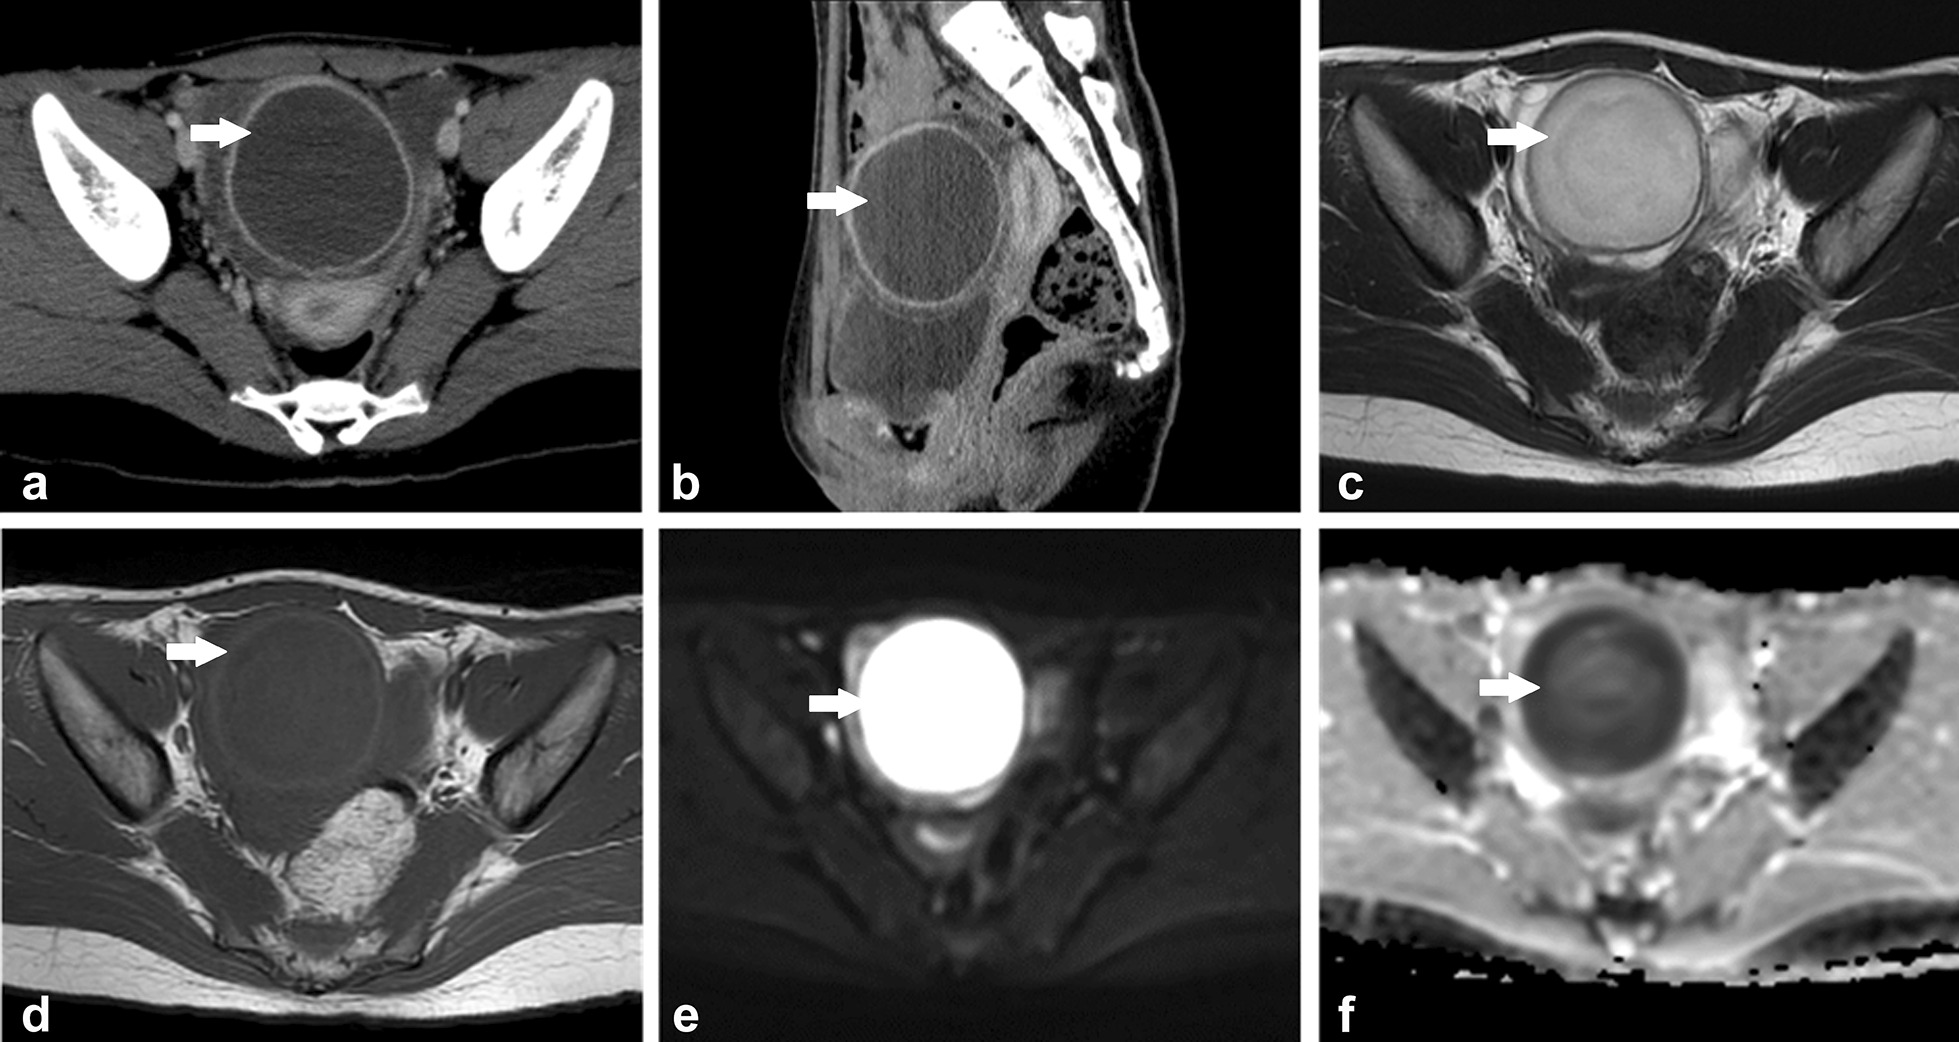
Computed tomography scan shows a unilateral and unilocular ovarian mass with dense fluid and a thick, uniform, enhancing wall in a transverse section image (a) and a sagittal image (b). Magnetic resonance imaging shows a right ovarian mass with intermediate signal intensity on T2-weighted image (c), low signal intensity on T1-weighted image (d), high signal intensity on diffusion-weighted image (e), and low diffusion, indicated by the apparent diffusion coefficient (f). The white arrow shows the abscess
